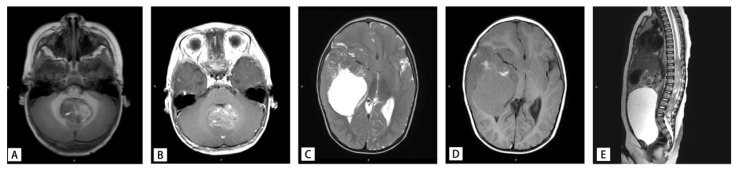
Atypical teratoid/rhabdoid tumor (AT/RT) magnetic resonance imaging. (A,B) Infratentorial tumor on T1-weighted imaging (T1WI) and enhanced T1-weighted imaging (T1WI). (C,D) Supratentorial tumor on T2-weighted imaging (T2WI) and T1-weighted imaging (T1WI). (E) Spinal cord tumor on T2-weighted imaging (T2WI).
